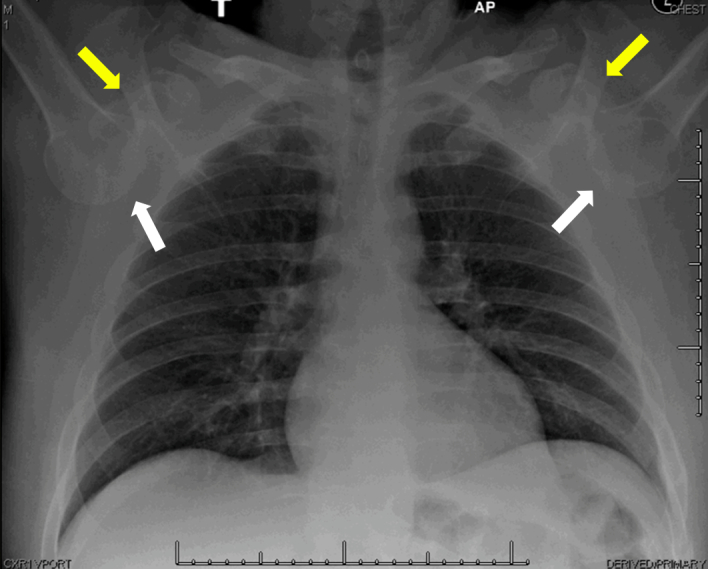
Radiograph of bilateral inferior shoulder dislocations. Humeral head (white arrow), displacement from glenoid (yellow arrow).
